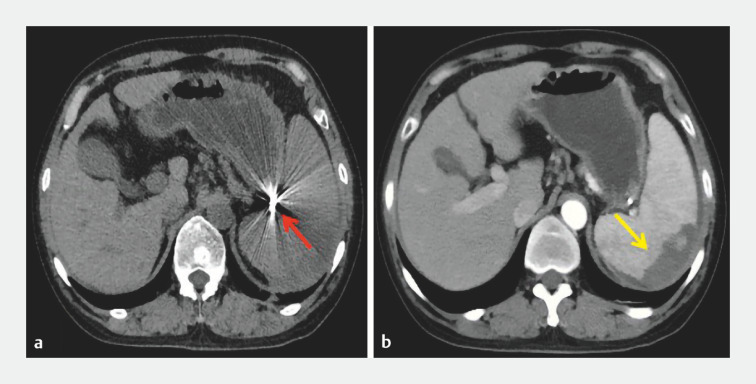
Enhanced computed tomography images showing:
